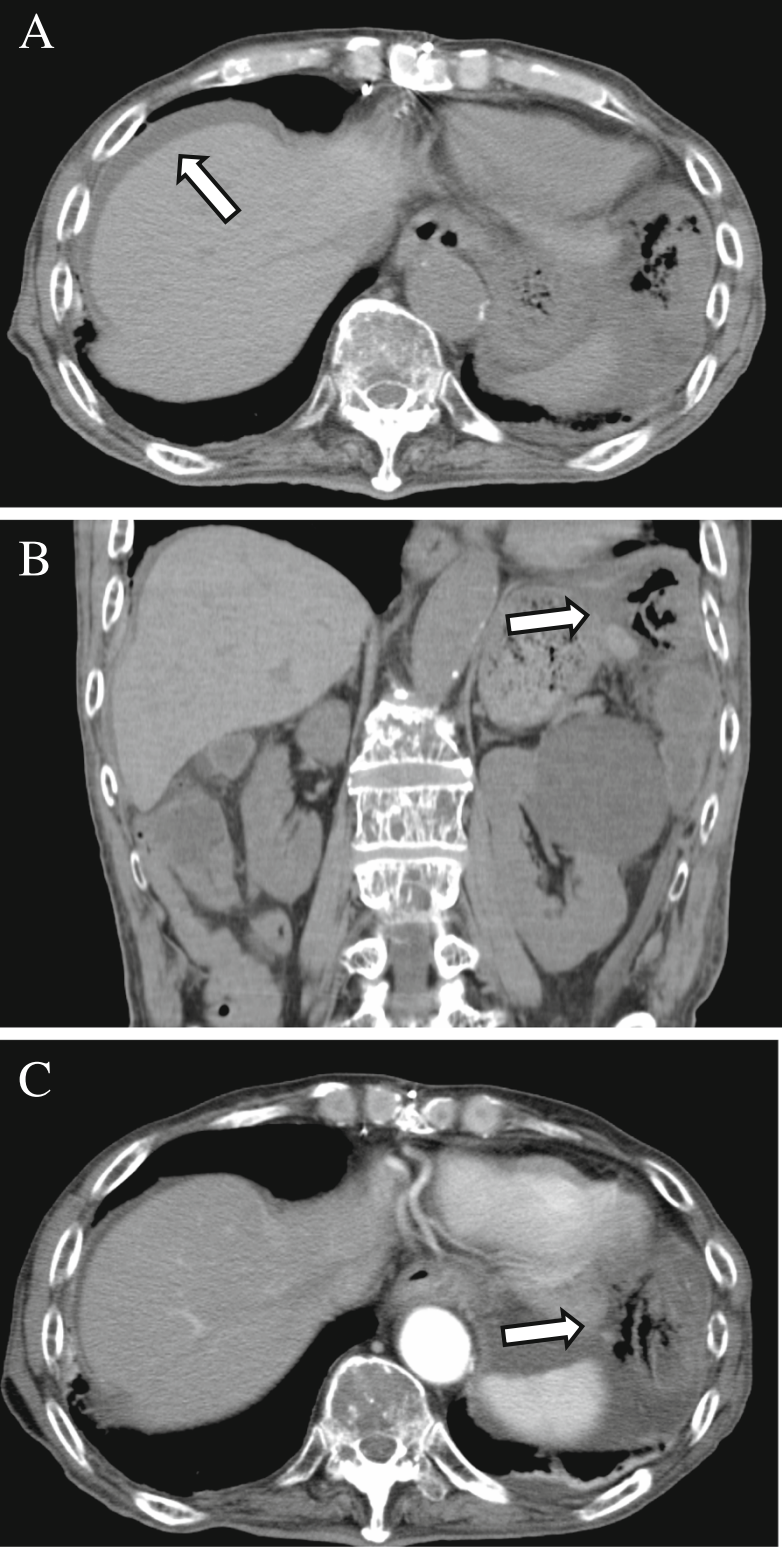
Abdominal computed tomography (CT). a Ascites around the liver (arrow). b, c Thickness in bowel wall and involving the free air around the colonic splenic flexure (arrow)
